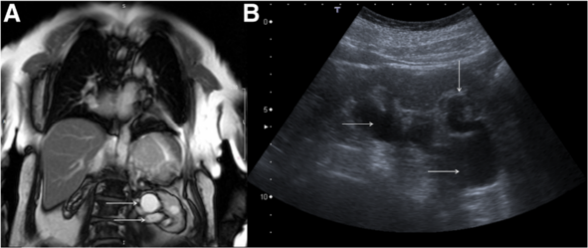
Coronal bright blood imaging (a) demonstrating a cystic entity at the left renal pelvis (solid white arrows). There is also a simple cortical renal cyst in the left lower pole. Correlative trans-abdominal ultrasound image (b) revealed the hypoechoic areas at the renal hilum are in continuity with a dilated proximal ureter and there is calyceal blunting (solid white arrows). These findings are consistent with hydronephrosis rather than para-pelvic cysts
